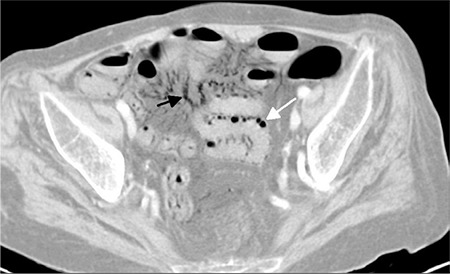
Contrast-enhanced abdominal computed tomography shows pneumatosis intestinalis (white arrow) and air in mesenteric vessels (black arrow)
